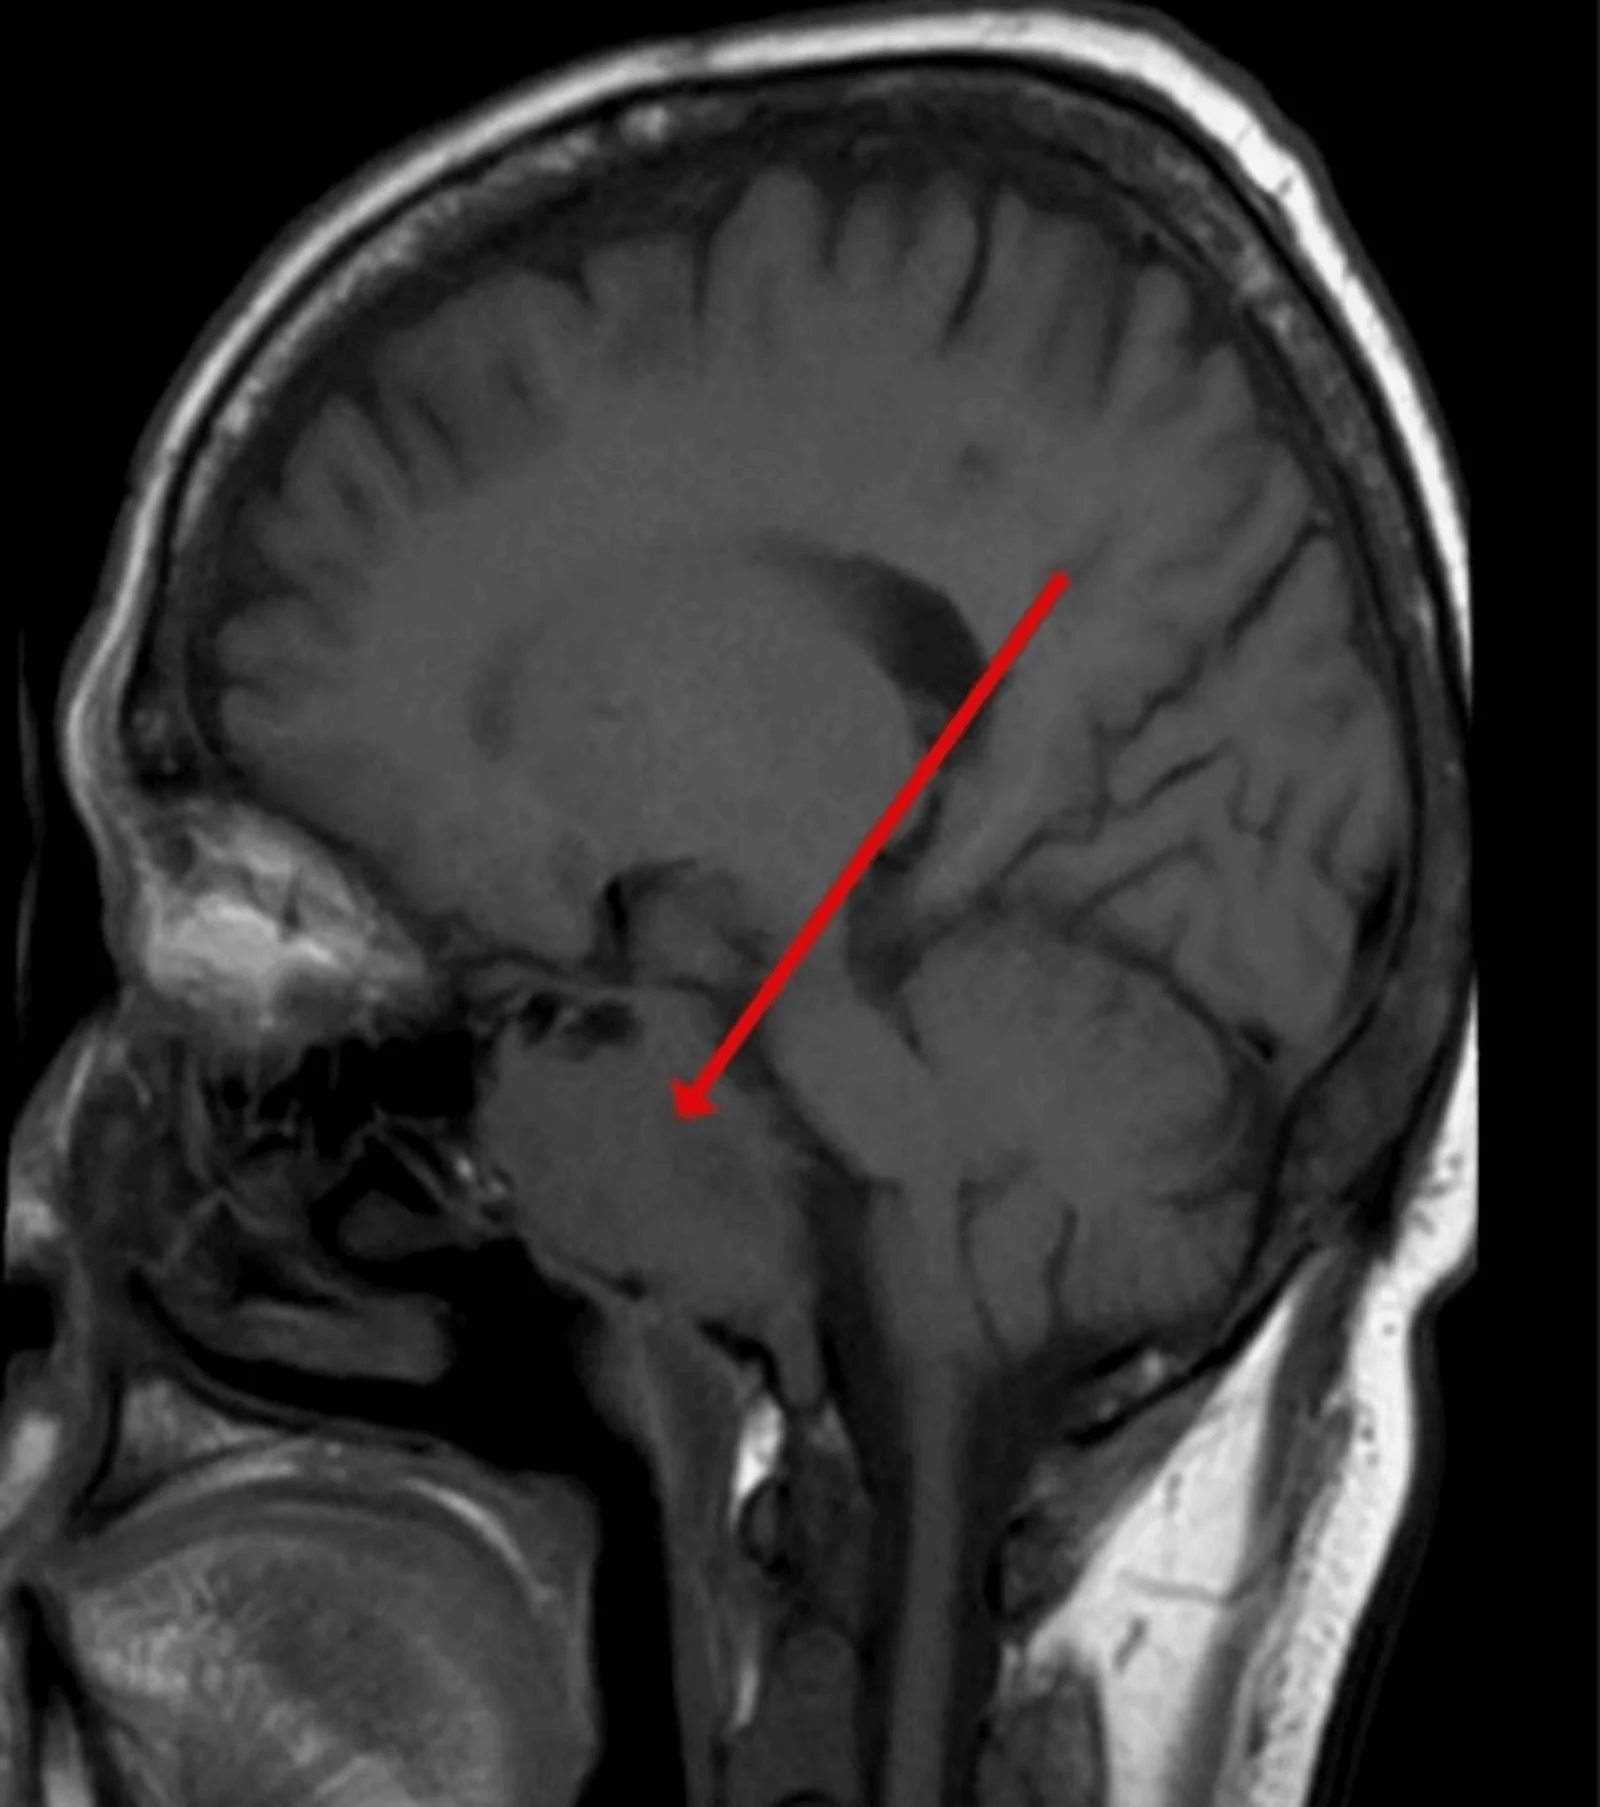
Orbital-brain MRI (clival mass with bone destruction and local spread) Expansive clival process with bone lysis and local extension, primarily suggesting a plasmacytic lesion given the context of multiple myeloma. Sagittal post-contrast T1-weighted image showing a large expansile process centered on the clivus (37.7 × 56 × 34.2 mm), isointense on T1/FLAIR, heterogeneously hyperintense on T2, without diffusion restriction, and enhancing after gadolinium injection. Local extension: superiorly, effacement of the sella turcica with probable invasion of the pituitary gland, pituitary stalk, and optic chiasm; on the left, invasion of the carotid canal and cavernous sinus, with 360° circumferential encasement of the left internal carotid artery; posteriorly, invasion of the left petrous apex; anteriorly, extension into the sphenoid sinus, more pronounced on the left. FLAIR: fluid-attenuated inversion recovery; MRI: magnetic resonance imaging
